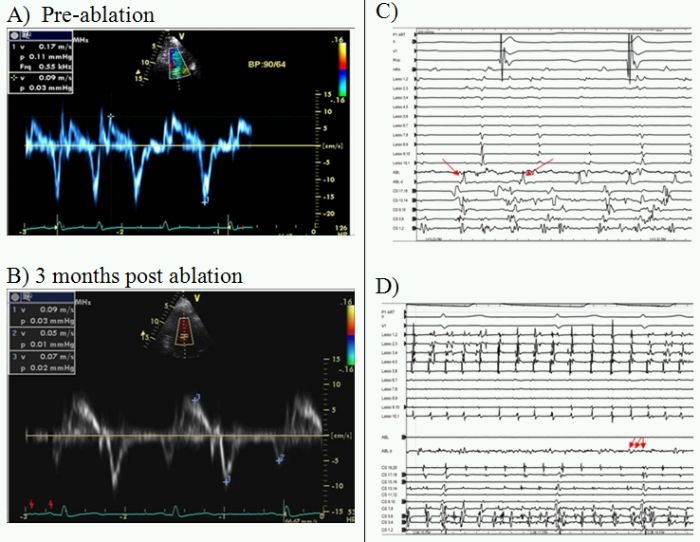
A pre- and 1B post-ablation tissue Doppler velocity tracings obtained on septal mitral annulus showing normal diastolic function (tissue Doppler e' > 10 cm/s).  The electrograms IC pre and post ID ablation show initial excellent amplitude electrograms (arrows) fragmented only with ablation suggesting fundamentally healthy atrial tissue as suggested by the normal tissue Doppler findings (primary electropathy).
